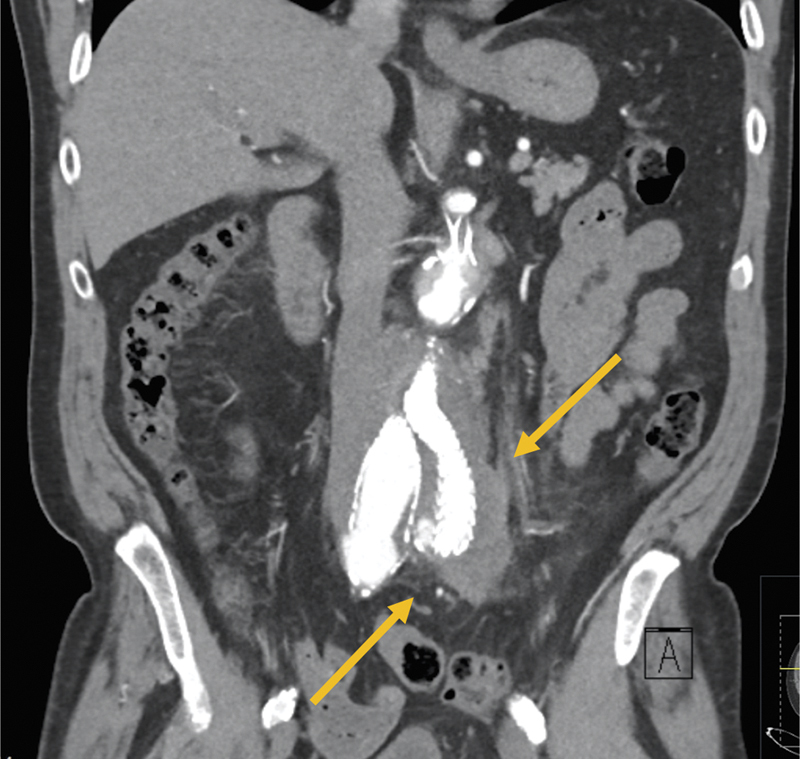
Coronal view of the preoperative contrasted computed tomography of the aorta, demonstrating the presence of a periaortic gas collection extending into the left iliac fossa (highlighted by yellow arrows).
